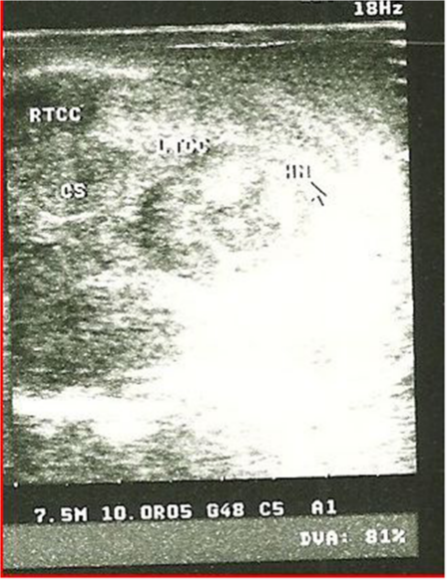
Penile ultrasound of the proximal third of the penile shaft. The penile ultrasound was performed an hour after presentation and shows a normal right corpus cavernosum, a ruptured left corpus cavernosum and associated hematoma. The corpus spongiosum appears normal.
